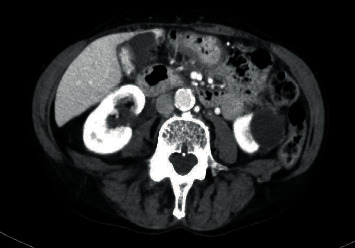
CT abdomen reported cholelithiasis with pericholecystic fluid.
